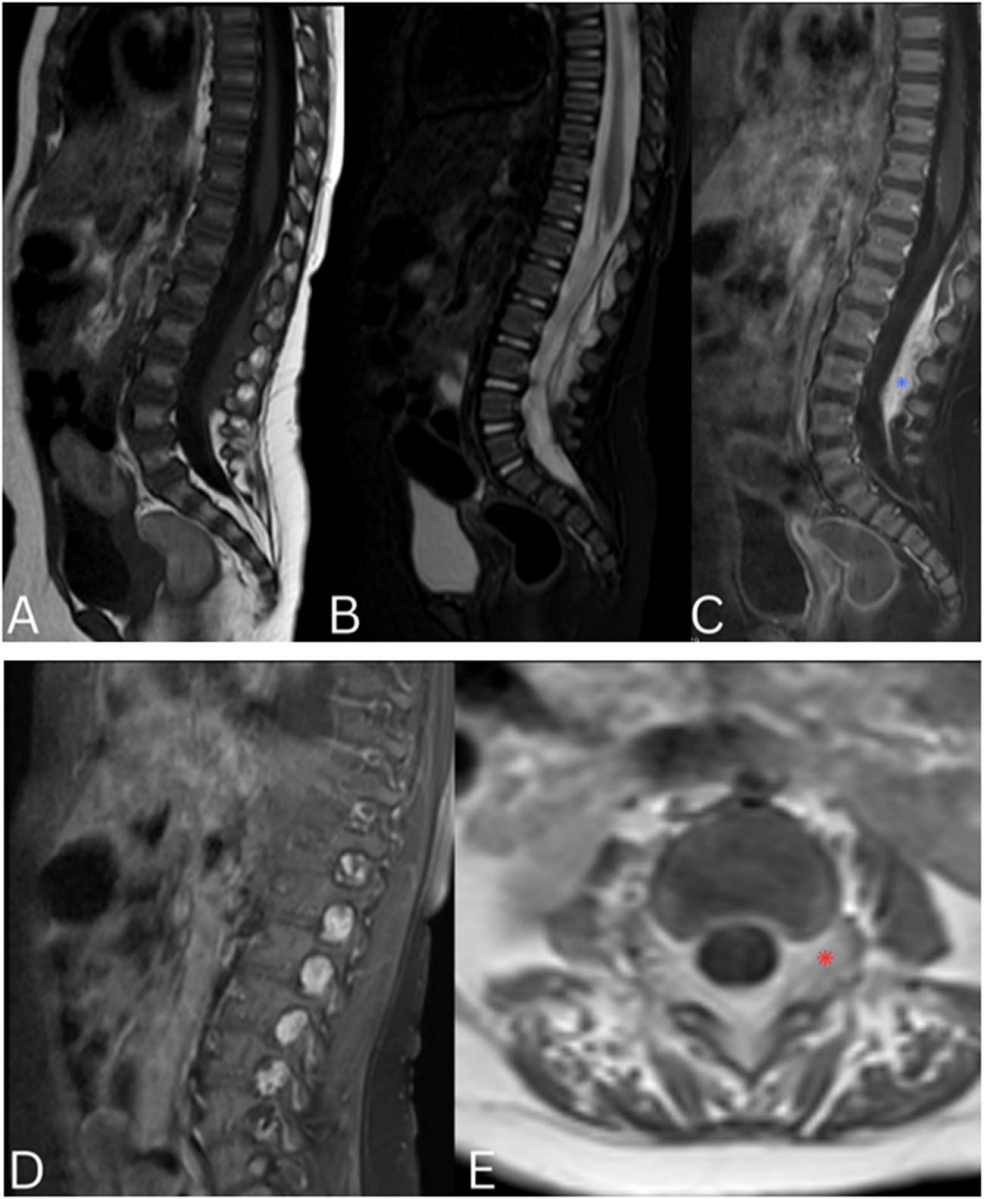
Sagittal T1 (image A), Sagittal T2 (image B), Sagittal T1 post gadolinium (image C and D), and axial T1 post gadolinium T2 (image E) images of the lumber spine 6 months after beta blocker therapy showing significant decrease in size and extent of the mass with residual posterior epidural component (blue asterisk, image C) with decrease but persistent areas of foraminal extension (red asterisk, image E). Not is made of significant decrease in the flow voids seen on prior MRI
